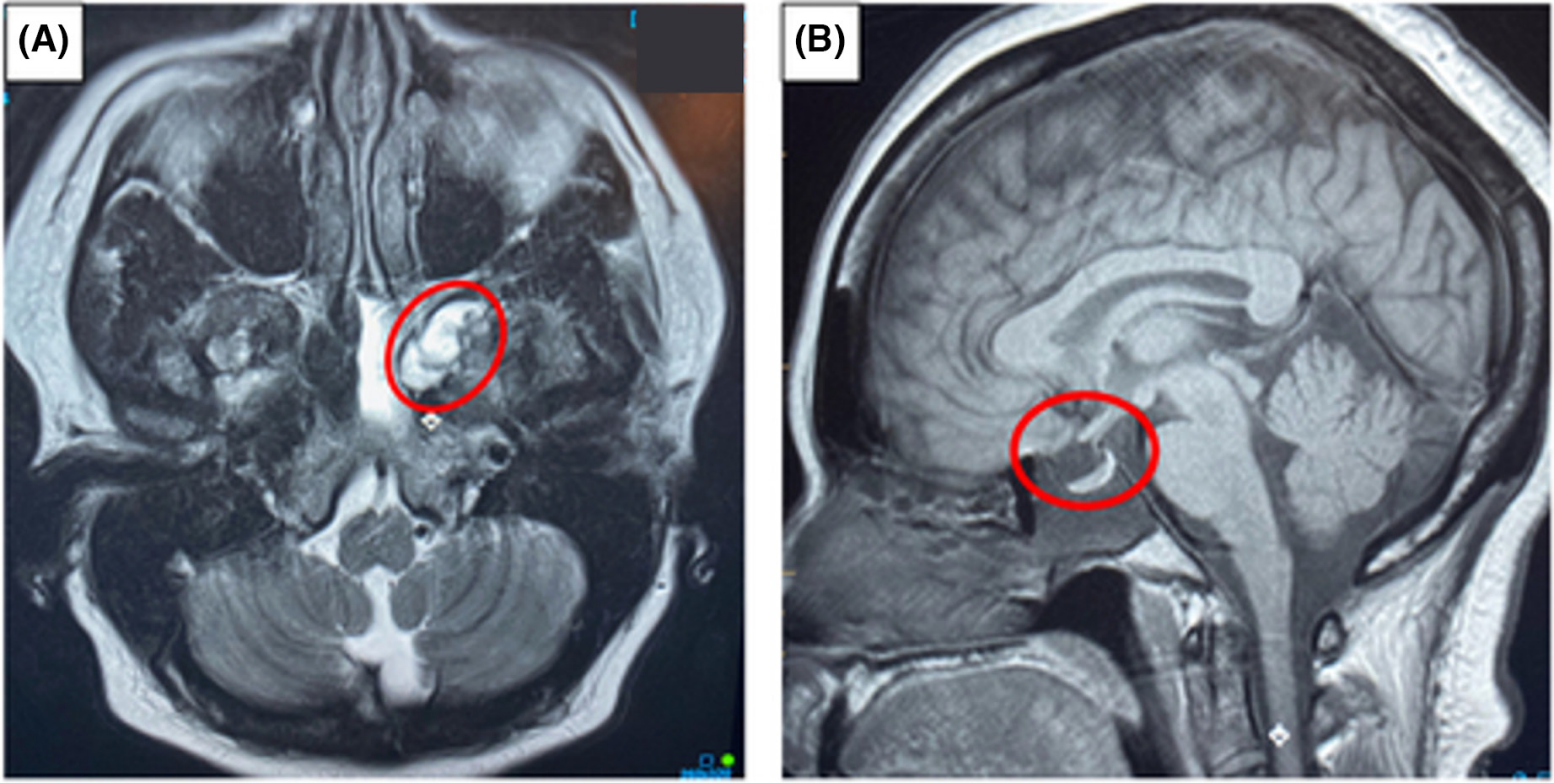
MRI of the skull base depicting (A) an encephalocele in the left sphenoid sinus (B) an empty sella (circled).
